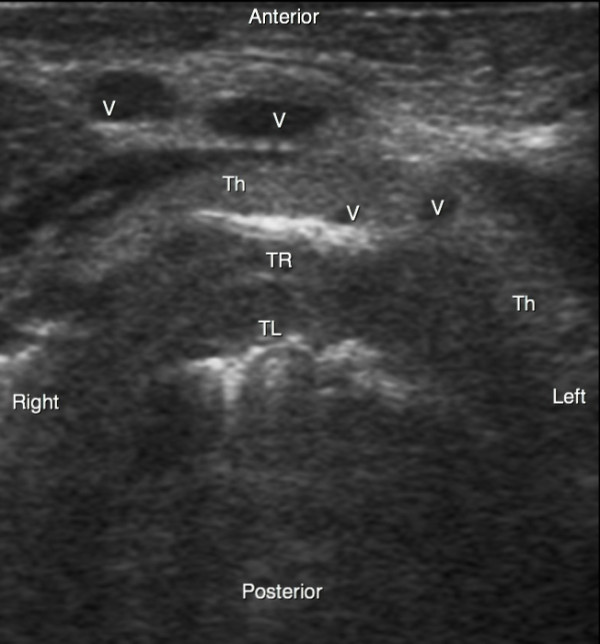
Ultrasound transversal view of the neck. Th, thyroid gland; TL, trachea lumen; TR, tracheal ring; V, vessel.
